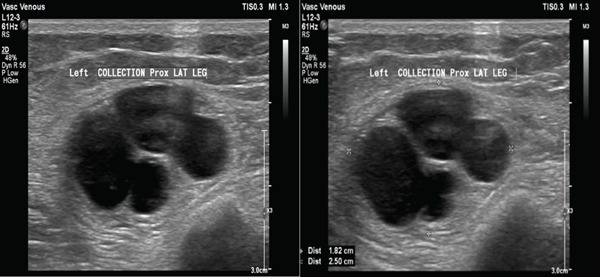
Ultrasound of the knee showing intramuscular complex tumor with cystic component and thick irregular septations measuring 7 × 2.6 × 2.1 cm.
